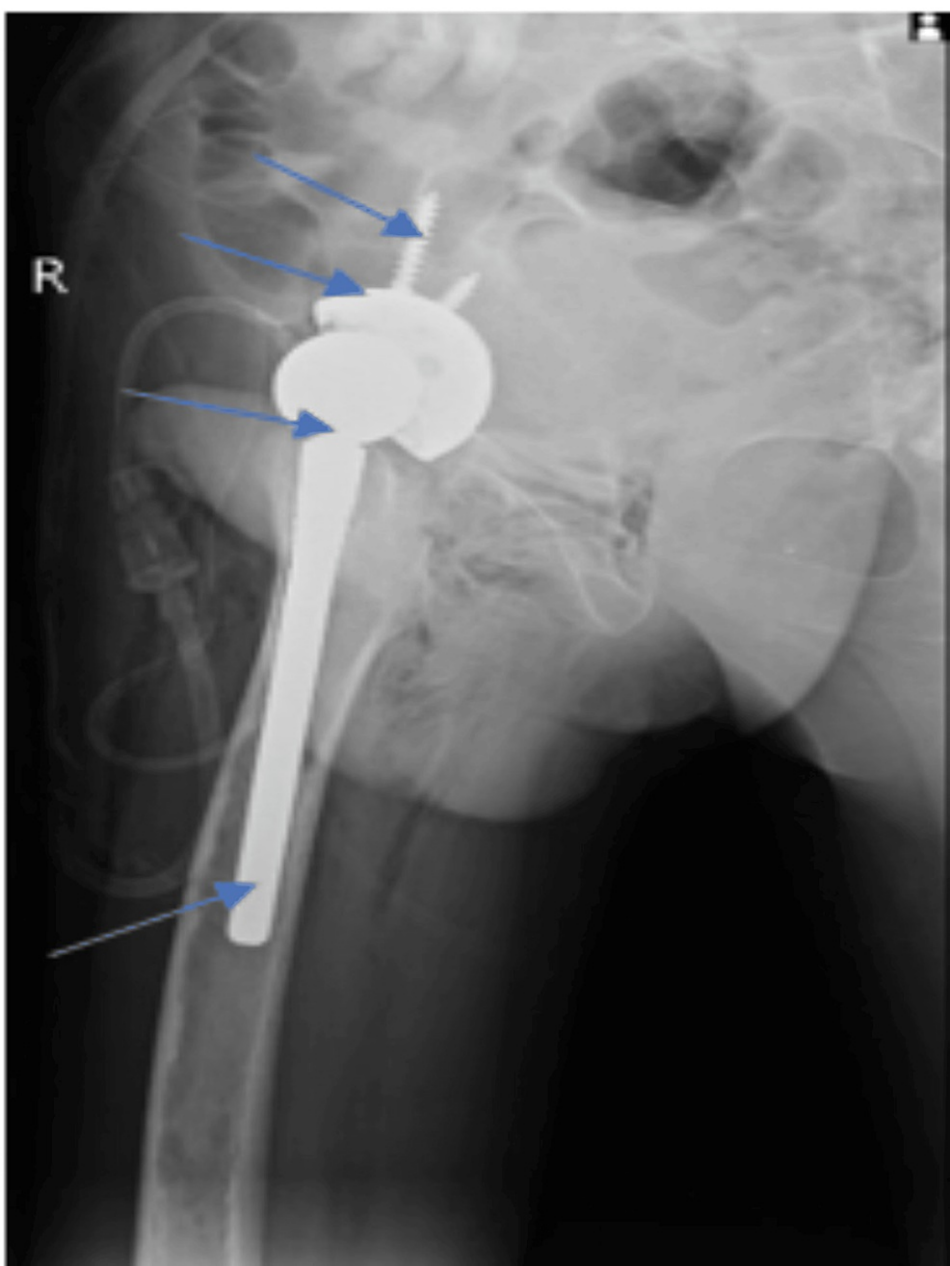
Postoperative X-rays of the uncemented total hip arthroplasty of the right hip joint showing a modular shell and hip component, bone screws, modular femoral head, and uncemented femoral stem.
